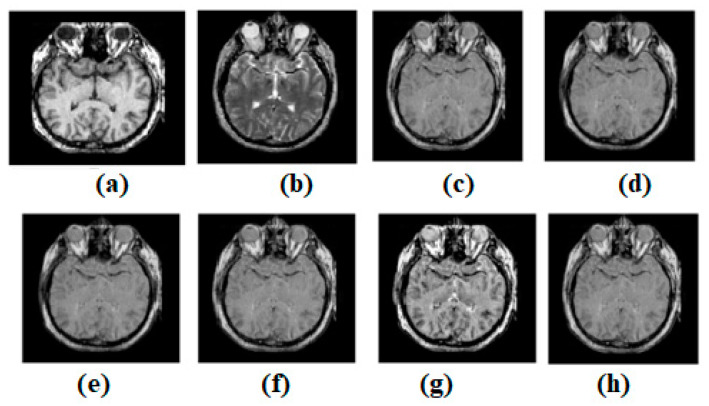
Multi-modality medical image fusion results (a) Source image: CT, (b) Source Image: MR, (c) Result of [44], (d) Result of [47], (e) Result of [48], (f) Result of [49], (g) Result of [51], (h) Result of [53].
